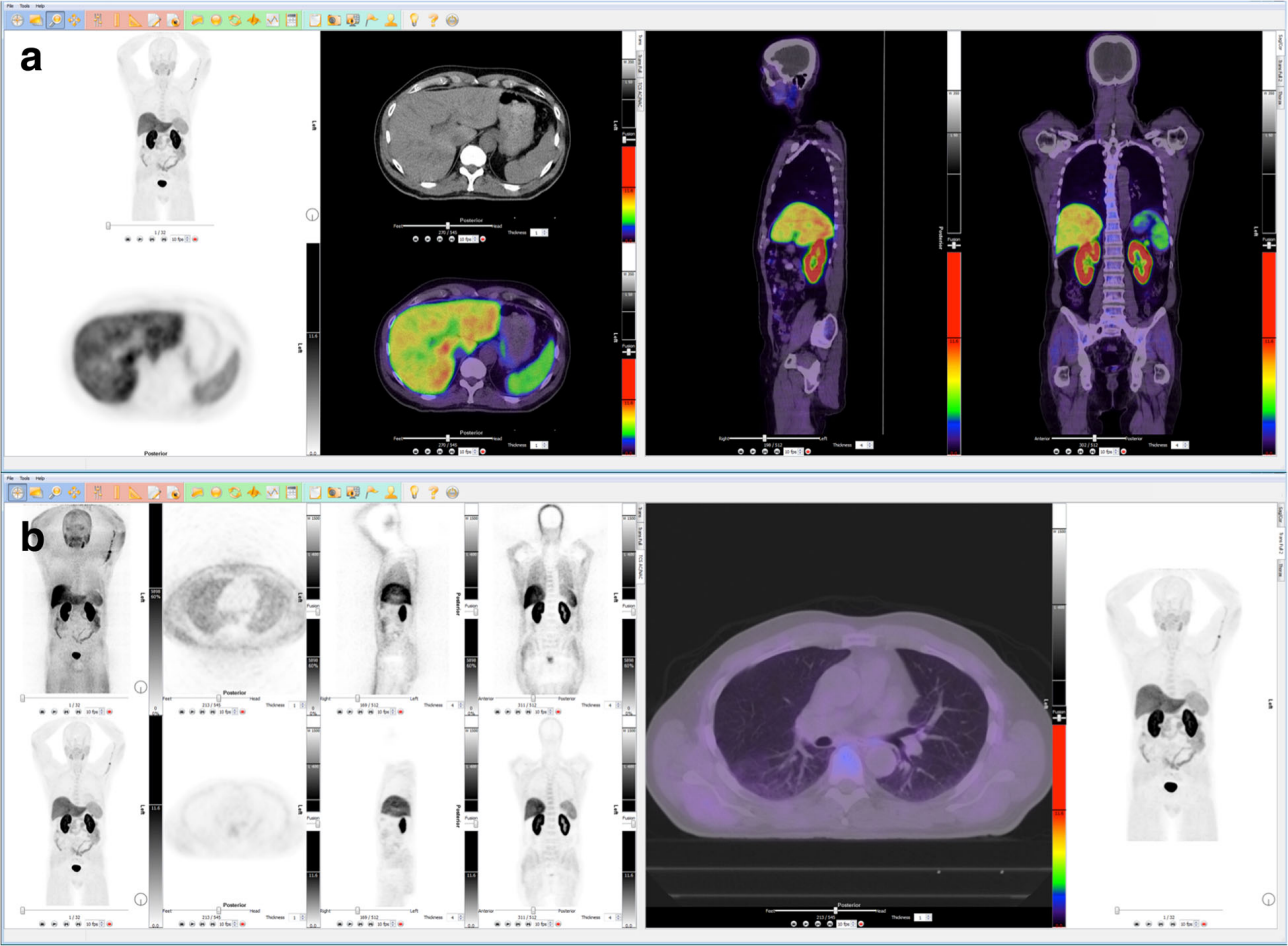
Dual-screen whole-body FCH-PET/CT image display template (Hybrid Viewer, Hermes Medical Solutions, Stockholm, Sweden). The primary tab (a) includes AC PET MIP plus CT, PET and fused PET/CT transaxial views on the left screen, and fused sagittal and coronal views on the right screen. The secondary tab (b) includes MIP and orthogonal views of NAC and AC PET on the left, and a large transaxial PET/CT view on the right. A rainbow color LUT (Siemens ECAT Rainbow) is used for the fused images, which are displayed with a linear, 50–50% blend. AC PET imaged intensity is normalized by adjusting the upper limit of the color scale so that the liver is nearly, but not, saturated (mostly yellow to orange). The same SUV upper threshold is used to adjust the inverse gray scale intensity of the PET-only images, as well as the dynamic PET images. This is a case of a negative FCH-PET/CT in a patient with biochemical relapse after radical prostatectomy, where physiological FCH distribution is seen
